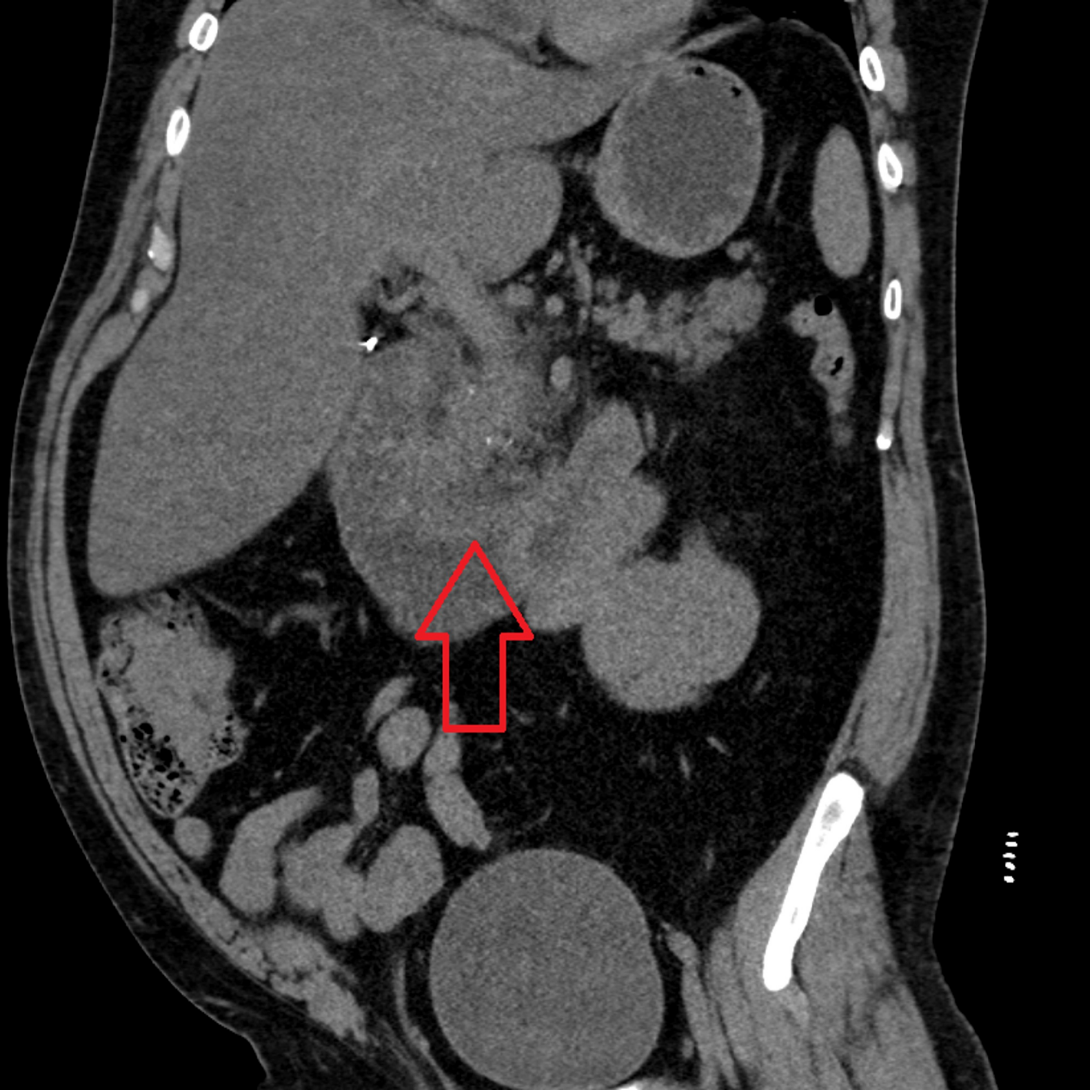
CT scan showing pancreatic fluid collection.
